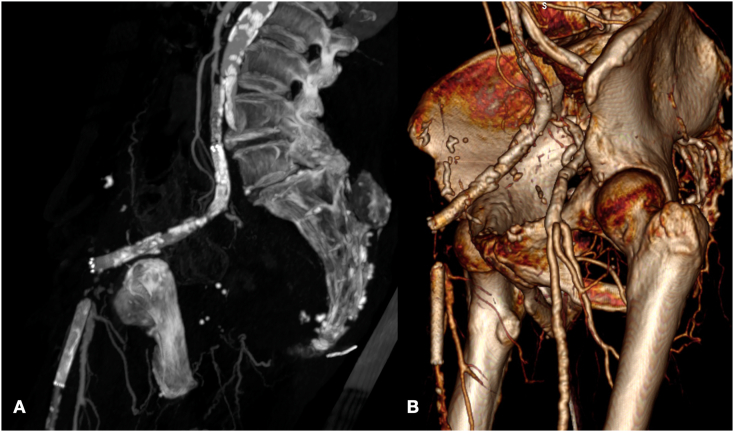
CT angiography MPR (A) and 3-dimensional volume rendering (B) before the second procedure.
